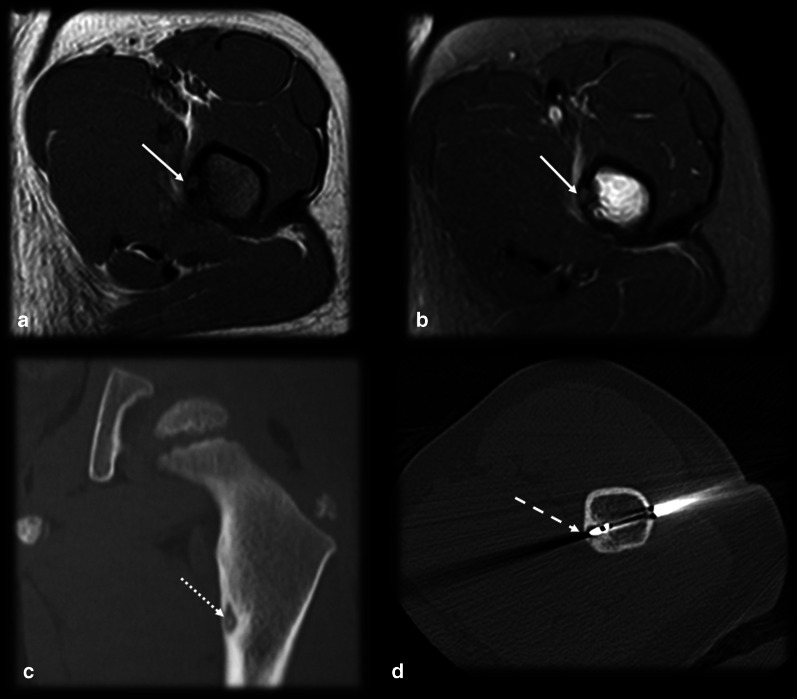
Typical osteoid osteoma and percutaneous ablation. Male, 15 years old, presenting with medial hip pain for 2 months, which became worse at night. Axial T1 (a) and T2 FS (b) MR images showing a small cortical nidus (arrows) within the femur shaft with a target-like appearance, edema and sclerosis. CT (c) better detected the partially mineralized nidus (dotted arrow), cortical thickening and sclerosis than did MRI. CT percutaneous biopsy and radiofrequency ablation (d) were performed (dashed arrow)
